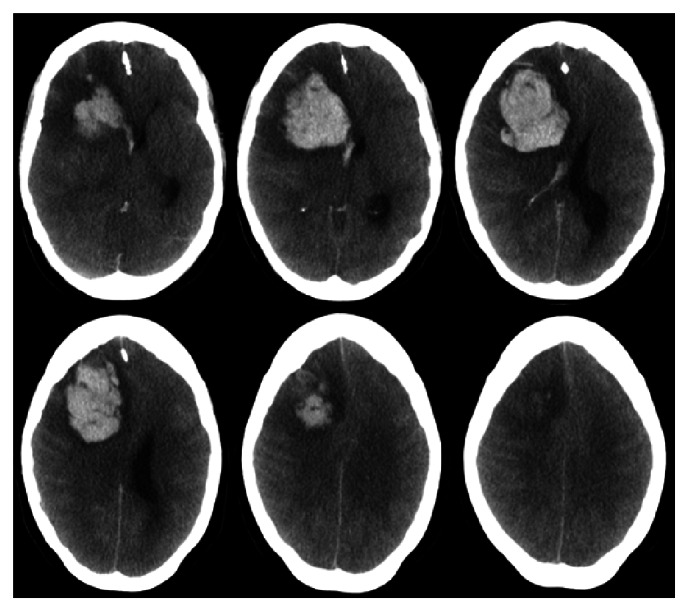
CT of the brain showing right frontal lobe intracerebral hemorrhage with massive brain edema, midline shift, and intraventricular extension.
